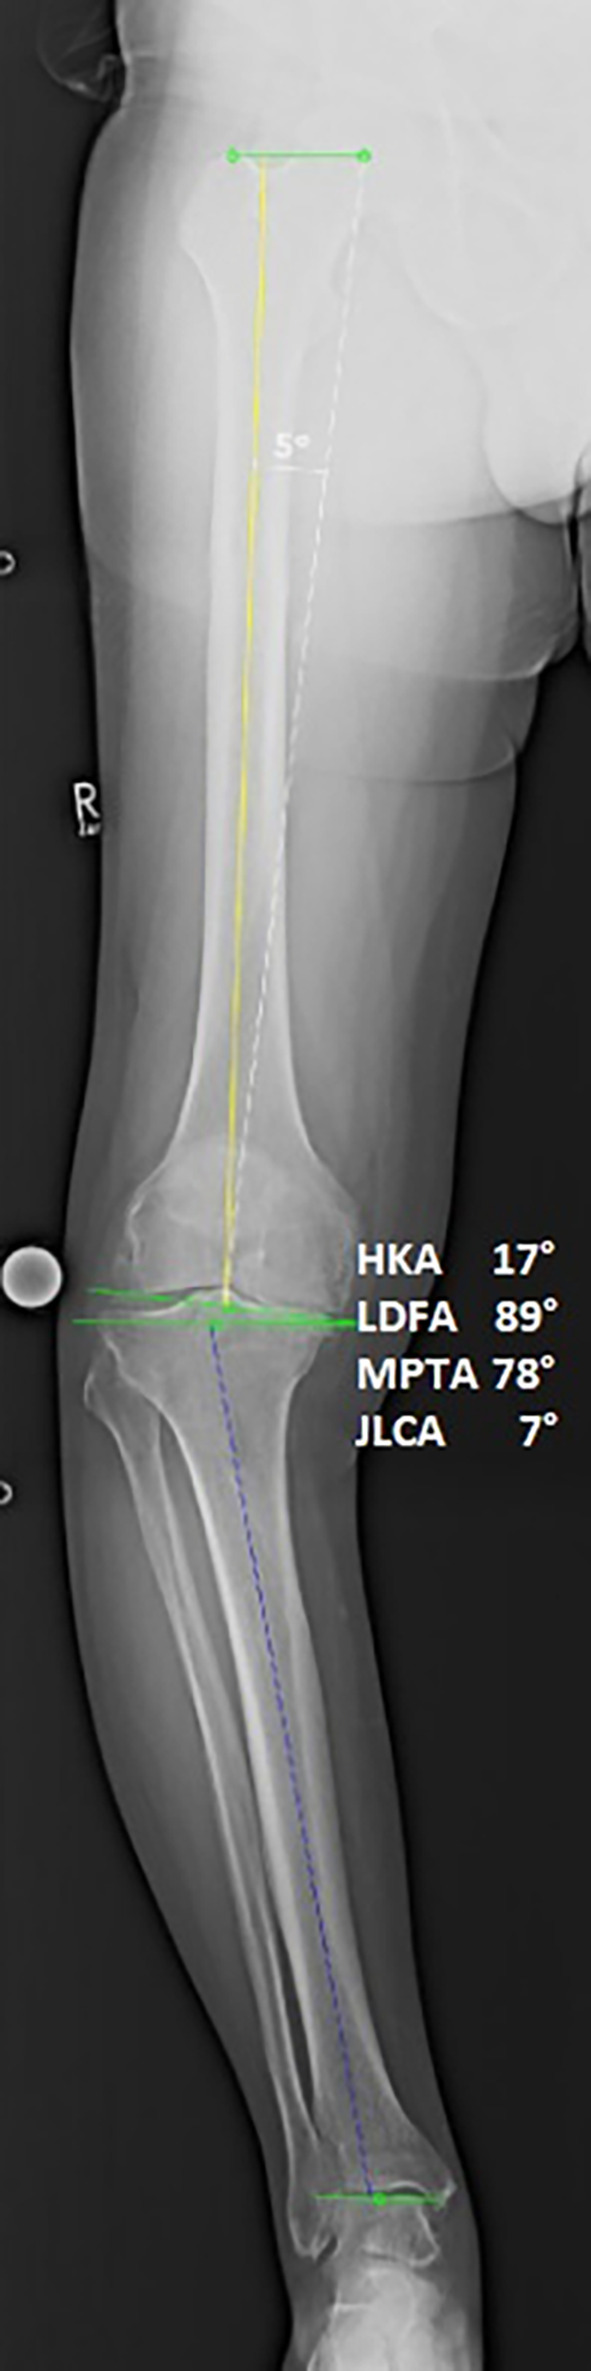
Preoperative full weightbearing long-standing anteroposterior radiograph of a varus knee with alignment references. HKA, hip-knee-ankle angle; JLCA, joint line convergence angle; LDFA, lateral distal femural angle; MPTA, medial proximal tibial angle.
